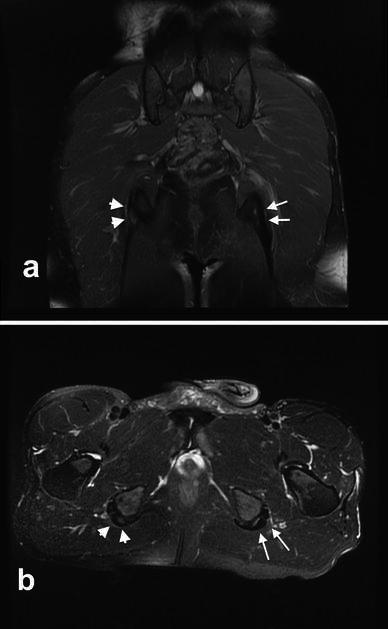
MRI of a 30 years old male long-distance runner 18 months after surgical treatment for left PHT. a Proton density-weighted coronal image showing no abnormal signal intensity of the left proximal hamstring insertion (*arrows*). The right proximal hamstring tendons present increased signal intensity compatible with PHT (*arrowheads*). b T2-weighted axial image clear shows no intratendinous structural abnormalities in the left proximal hamstring tendons (*arrows*). The right side presents signs of PHT (*arrowheads*). This patient underwent surgical treatment for right PHT four months after having performed the MRI, but he was not considered in this study because of the follow-up inferior to 24 months
